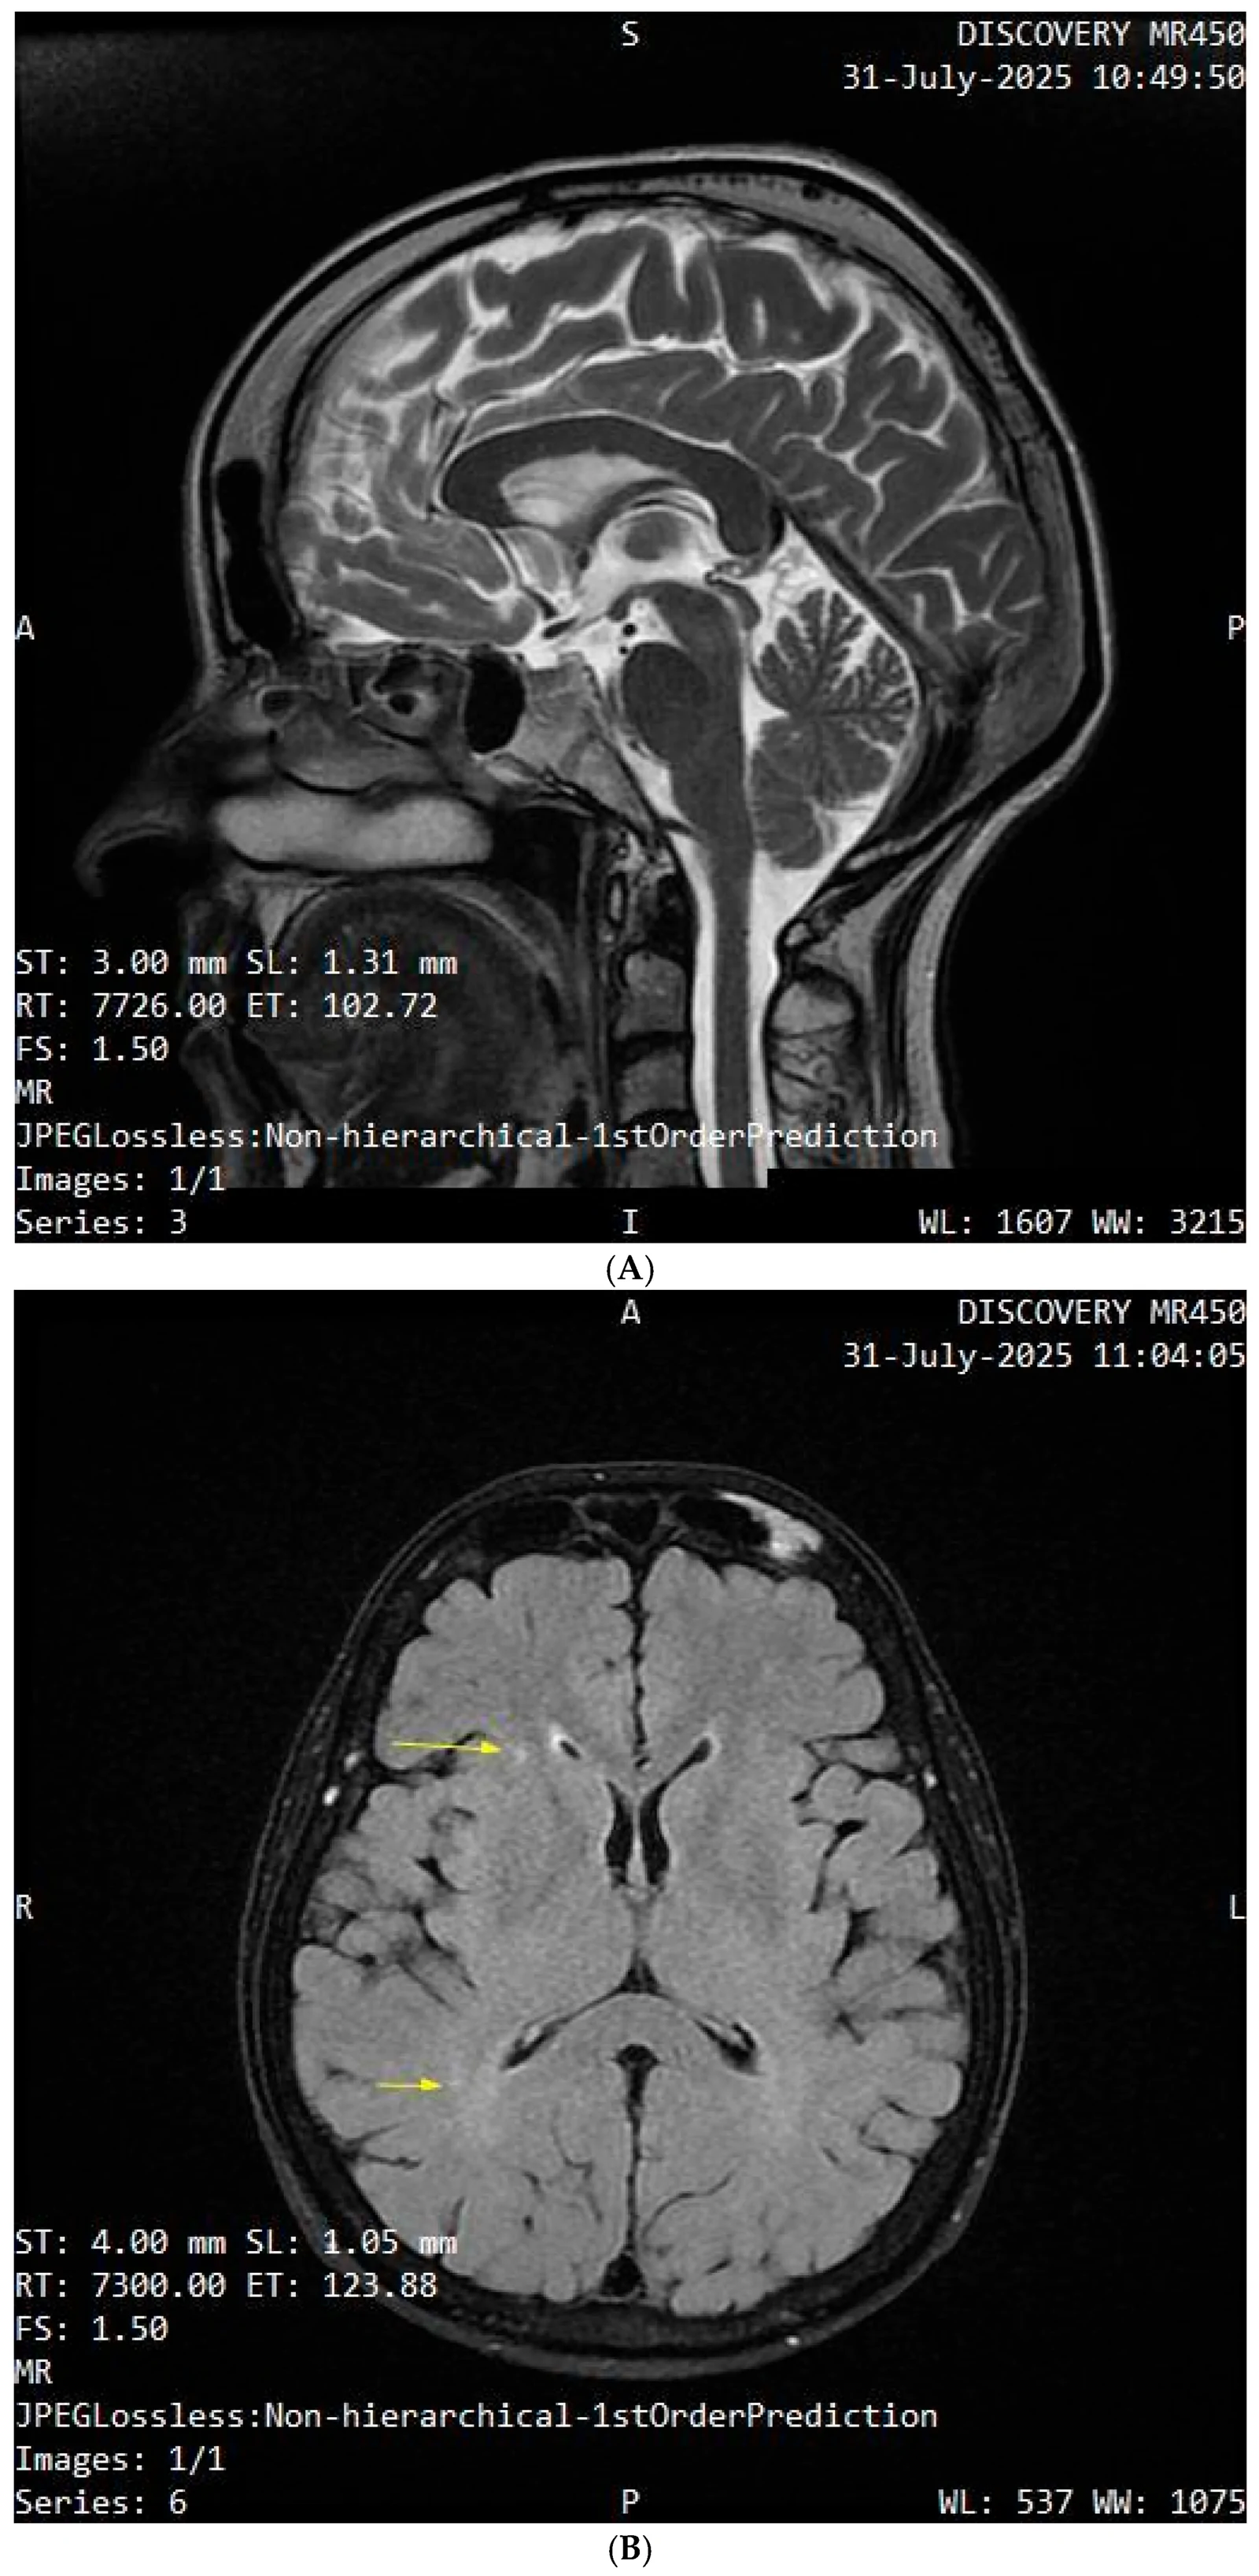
Initial brain magnetic resonance imaging (MRI, 1.5 T) performed on 31 July 2025. (A) Sagittal T2-weighted image demonstrating normal anatomical structures of the brainstem, fourth ventricle, and cerebellum without evidence of atrophy or focal lesions in the posterior fossa. (B) Axial T2-FLAIR image at the level of the lateral ventricles revealing isolated small hyperintense foci in the subcortical white matter (yellow arrows), consistent with mild chronic small-vessel ischemic changes (Fazekas grade 1), and moderate generalized cortical atrophy (GCA grade 2). No evidence of mass effect, diffusion restriction on DWI, microbleeds on SWI, or pathological brain contrast enhancement was observed.
